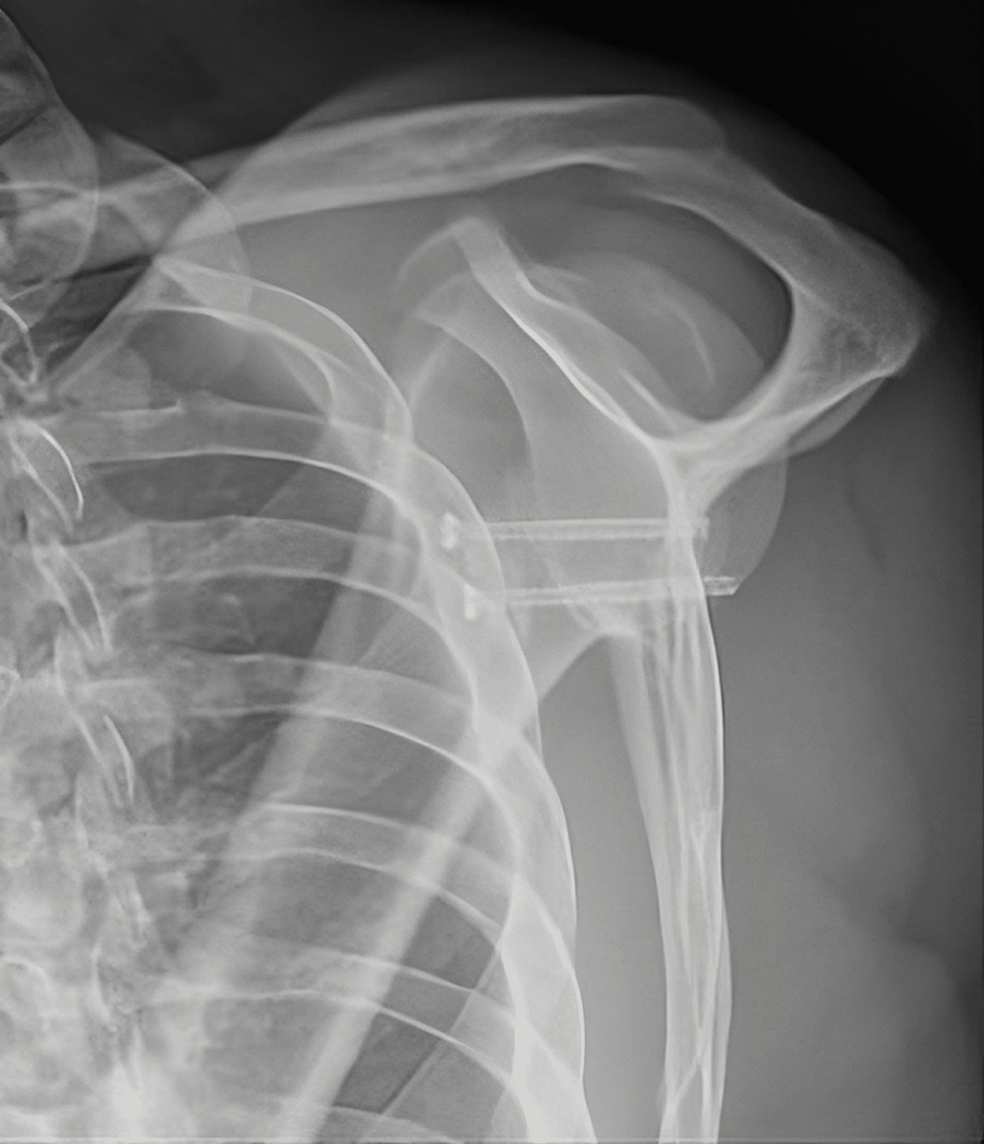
Postoperative radiograph (scapular Y view) showing left shoulder Latarjet procedure.
